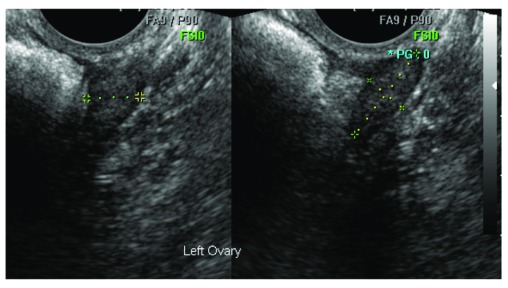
Transverse (TS) and longitudinal (LS) transvaginal ultrasound images of left ovary acquired by sonographer. This ovary was confirmed as normal and correctly measured by the expert reviewer.
